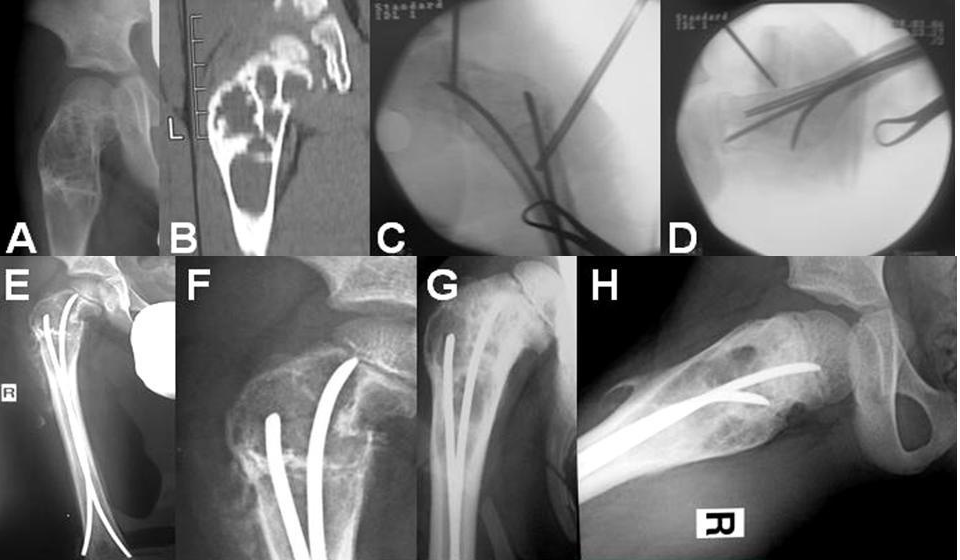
(A) Plain radiograph and (B) computed tomography scan of a 6 year-old boy with a simple bone cyst at the right proximal femur. (C and D) Intramedullary nailing and injection of demineralized bone matrix and autologous bone marrow from the ipsilateral iliac crest was done.(E and F) Plain radiographs of the right proximal femur show a subcapital femoral neck fracture after a fall from a bike three weeks postoperatively. (G) Anteroposterior and (H) lateral radiographs of the right proximal femur nine months postoperatively, show thickening of cortex and healing of the cyst (Neer stage II).
